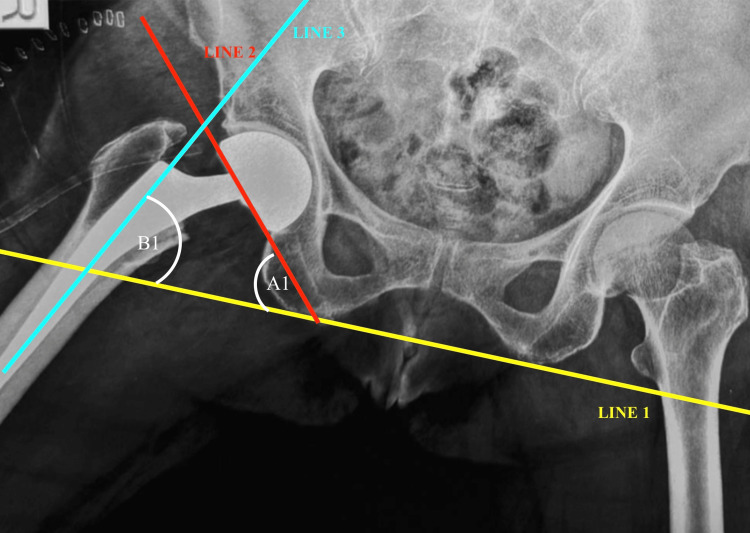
AP pelvic radiograph in maximum abduction showing corresponding angular measurements (A₁ and B₁) The same technique was performed on the maximal abduction anteroposterior radiograph, with the angles denoted as A1 and B1. AP, anteroposterior
